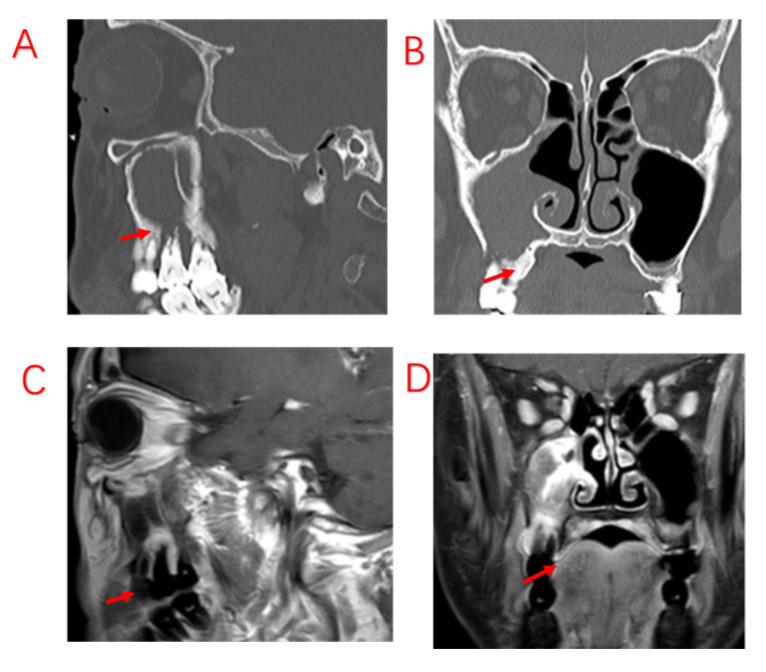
Patient with severe periodontal infection and no improvement in sinusitis symptoms after ESS. (A,B): Sinus CT shows adequate maxillary sinus opening, alveolar bone resorption of the diseased tooth, and bone defects in the MSF. (C,D): MRI shows periodontal lesion tissue attached to the mucosa of the maxillary sinus, with significant swelling of the maxillary sinus mucosa. Red arrows indicate specific sites of bony defects in MSF.
